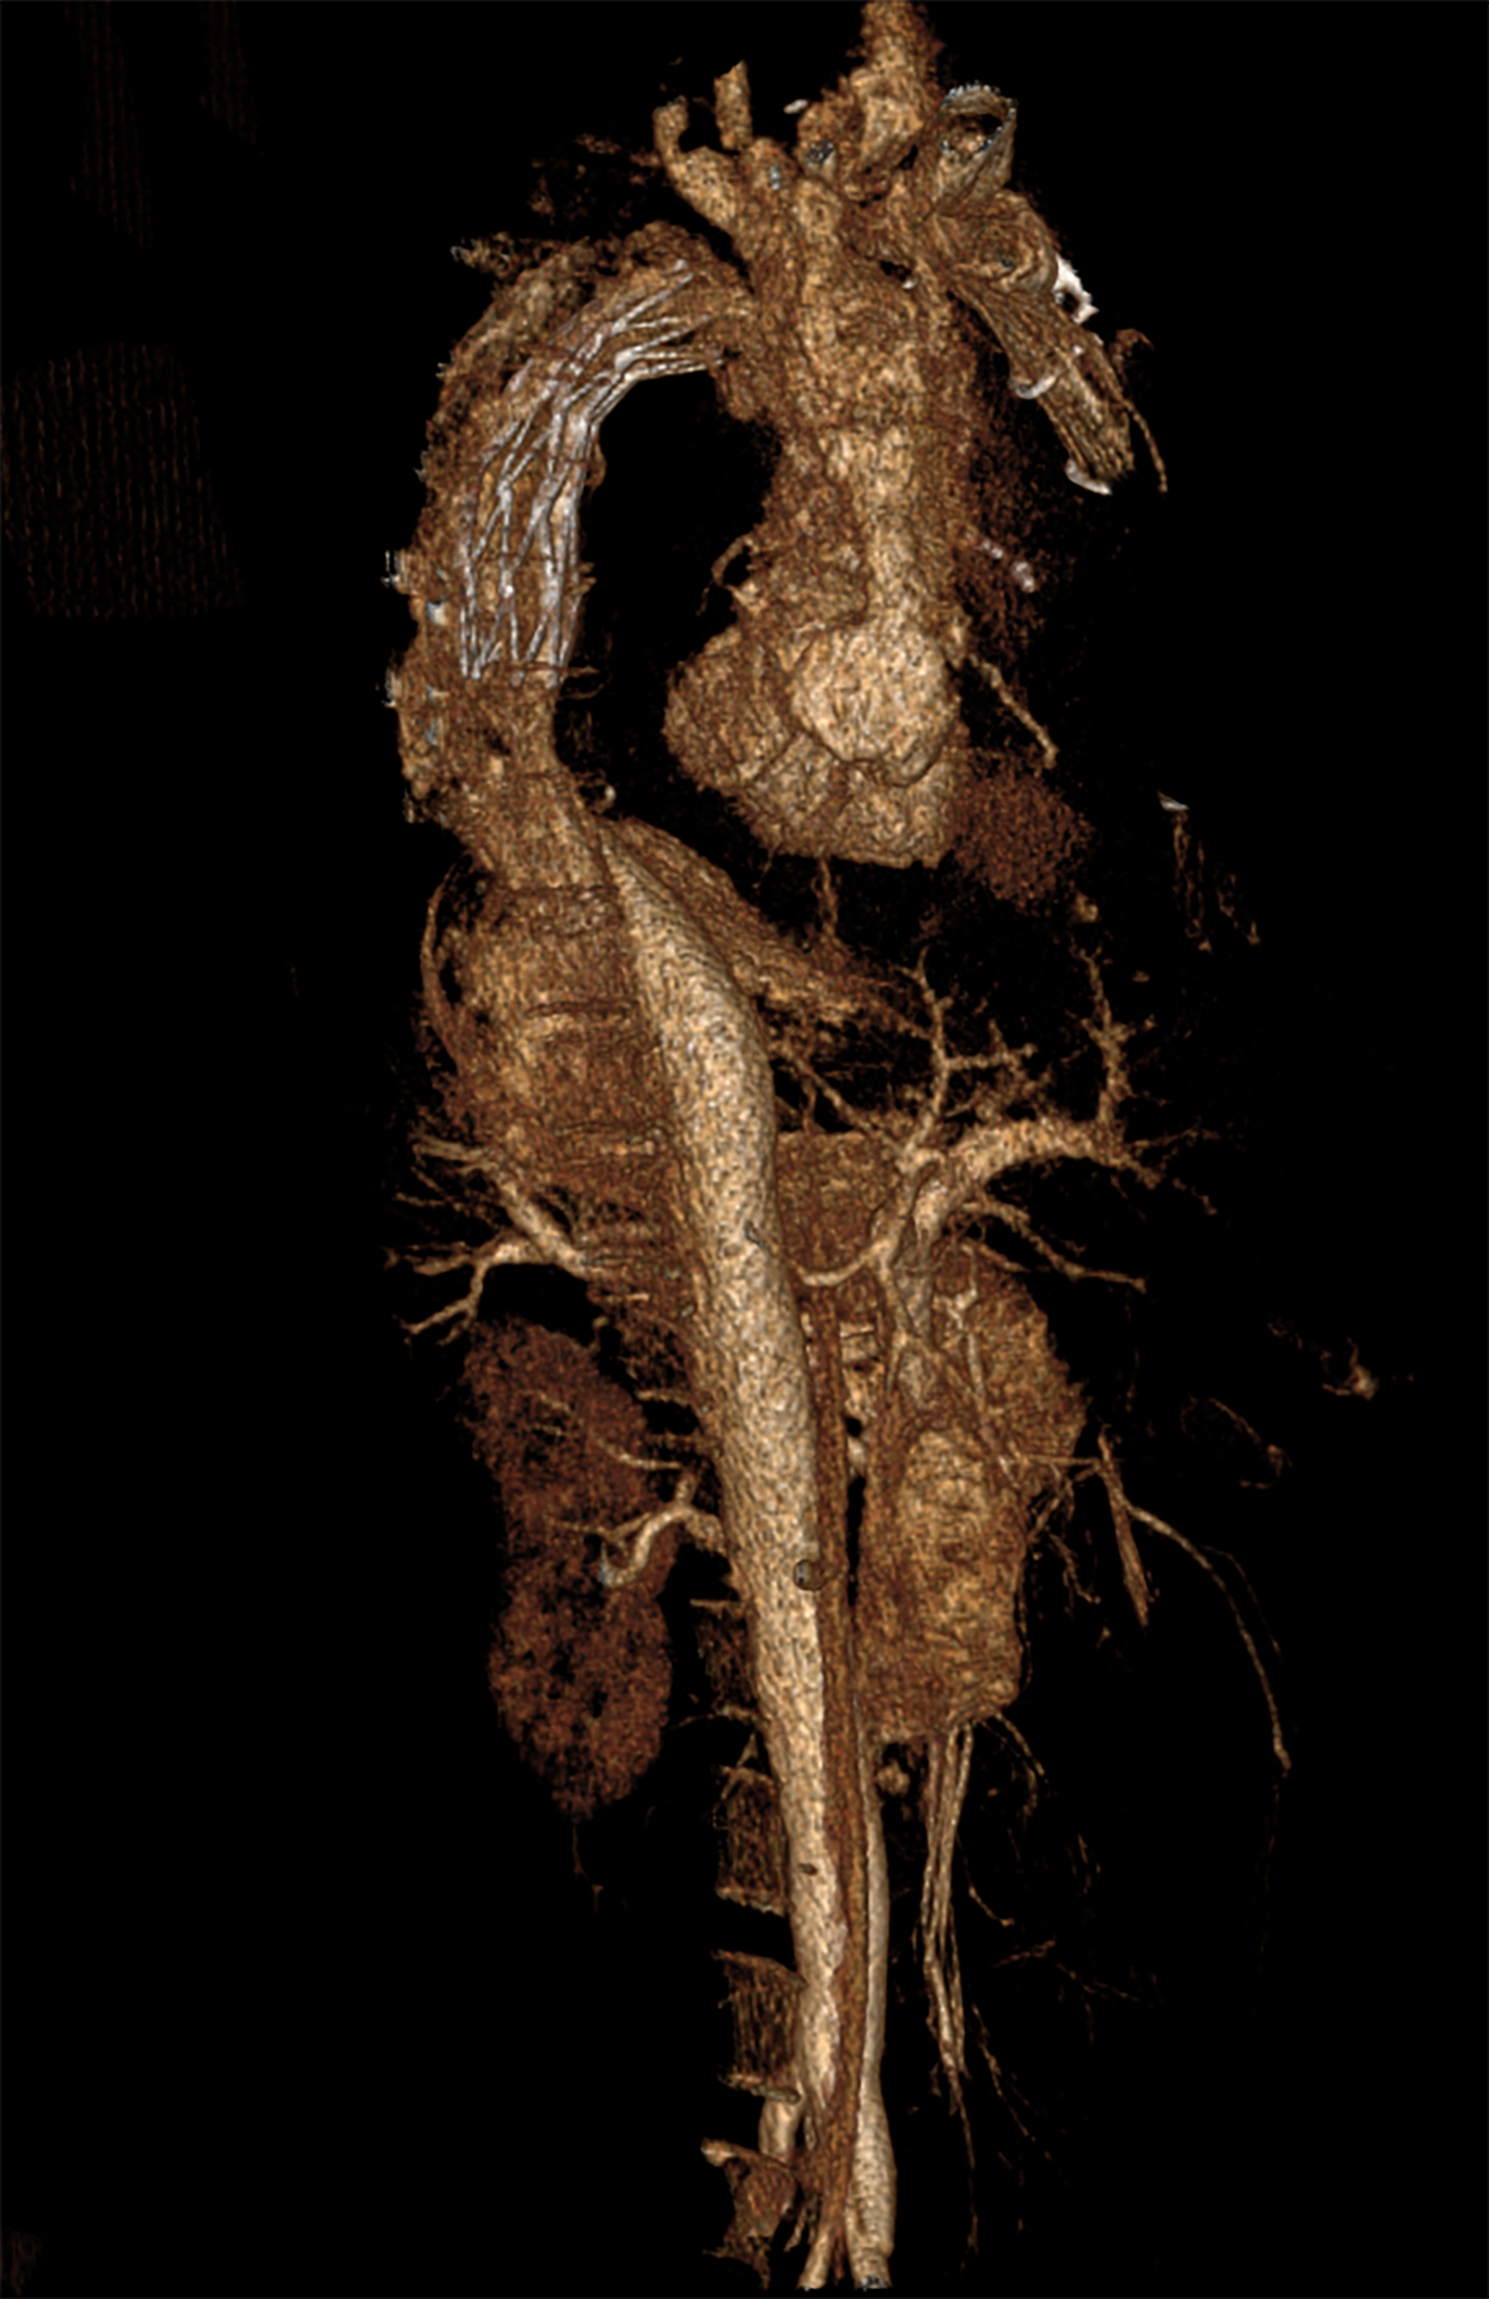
Radiological findings. Three-dimensional reconstruction of the postmortem computed tomography angiography (PMCTA) findings using a VRT. The image shows that the tiny blood vessels of the organs and tissues were filled with contrast medium and no contrast agent flowed from the blood vessel
